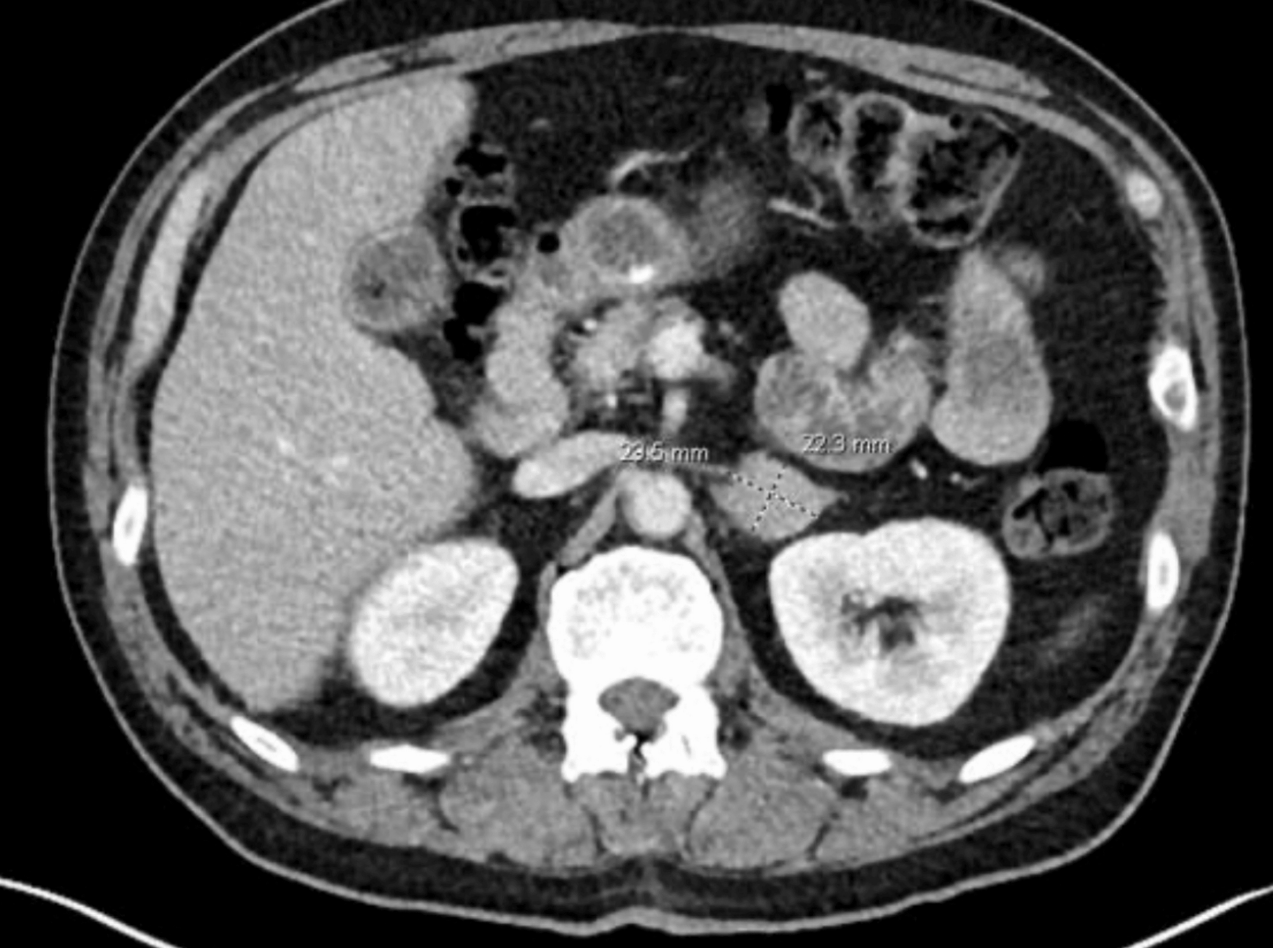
Axial section of CT abdomen with intravenous contrast showing a 2.2 x 2.4 cm left adrenal mass.
